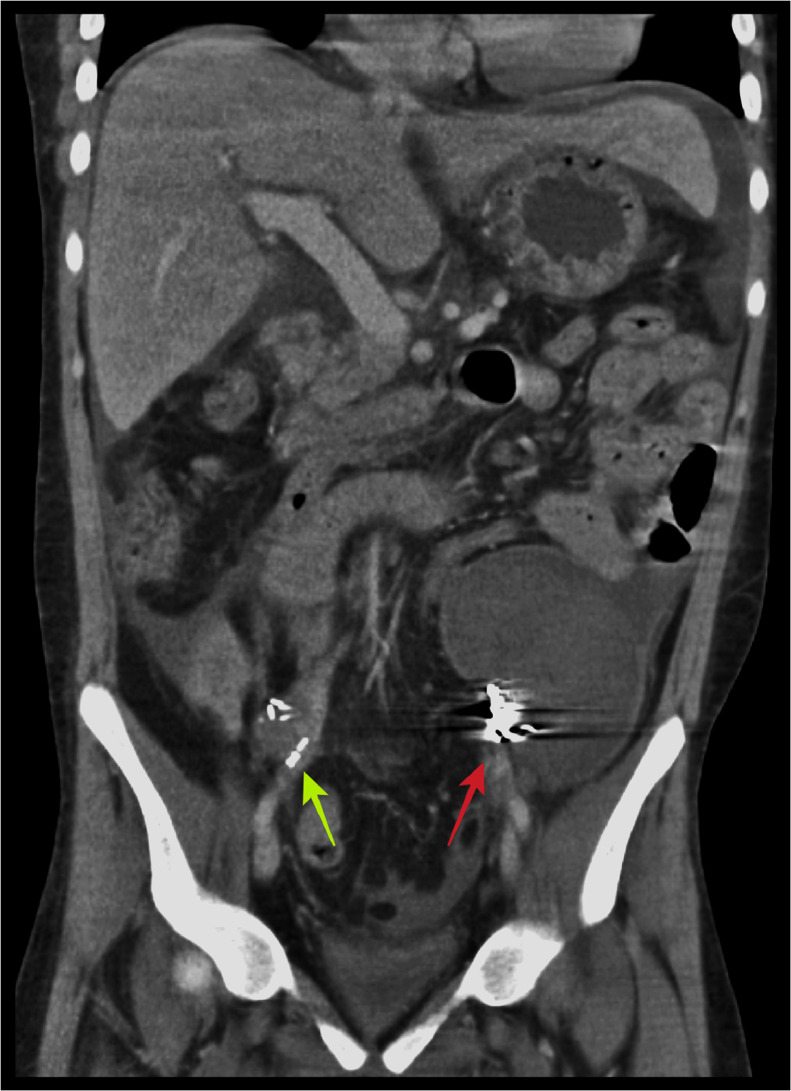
Coronal CT imaging of the patient's abdomen and pelvis following embolization of the left renal allograft. Red arrow highlights the embolization coils from the left renal graft embolization. The left renal graft remains swollen, edematous, and without enhancement except for a slight degree of enhancement at the cortical rim, due to proximal perforators supplying the cortex. The overall graft has structural distortion from necrosis. Green arrow identifies the surgical clips from the pancreatic implant, overlying the right iliac artery.
